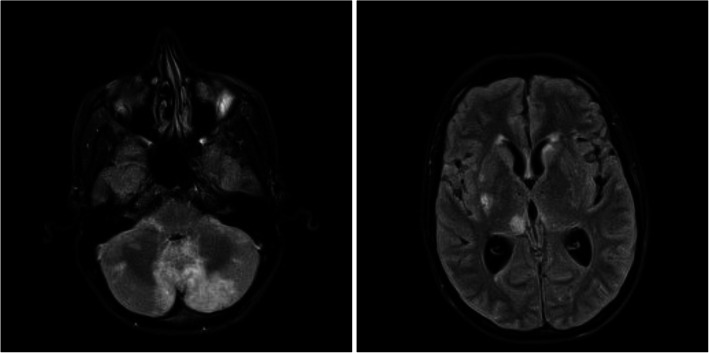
MRI Brain case 2 with multiple acute infarctions in cerebrum, brainstem, and cerebellum read as likely secondary to septic embolic
